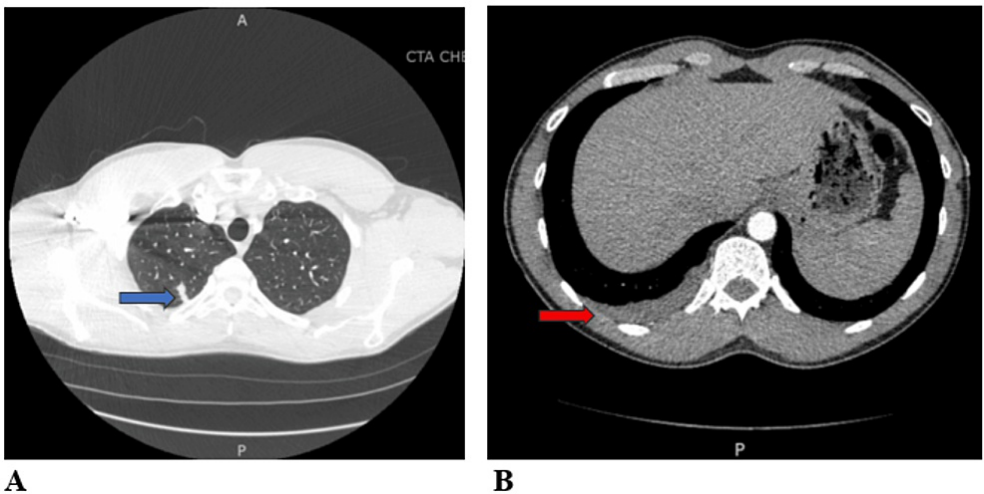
CT chest without contrast shows a cluster of tree-in-bud nodules in the right lower lobe, a new right upper lobe peripheral bandlike nodule, and a small right pleural effusion. The blue arrow indicates the right upper lobe peripheral bandlike nodule. The red arrow indicates the small right pleural effusion. (A) The lung window shows the right upper lobe peripheral bandlike nodule (blue arrow). (B) The soft tissue window shows a small right pleural effusion (red arrow).
